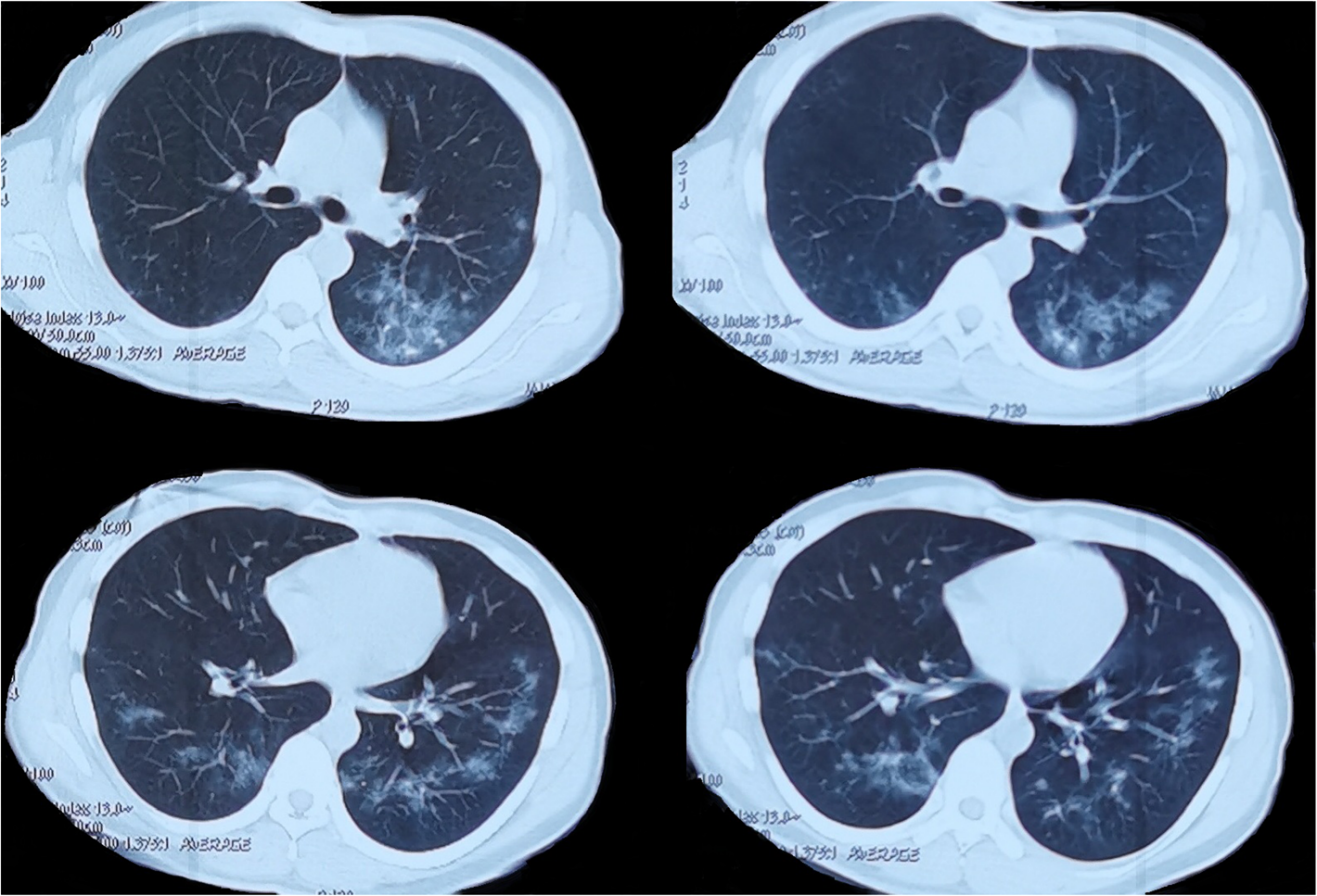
Chest CT images of patient one on Feb 9
